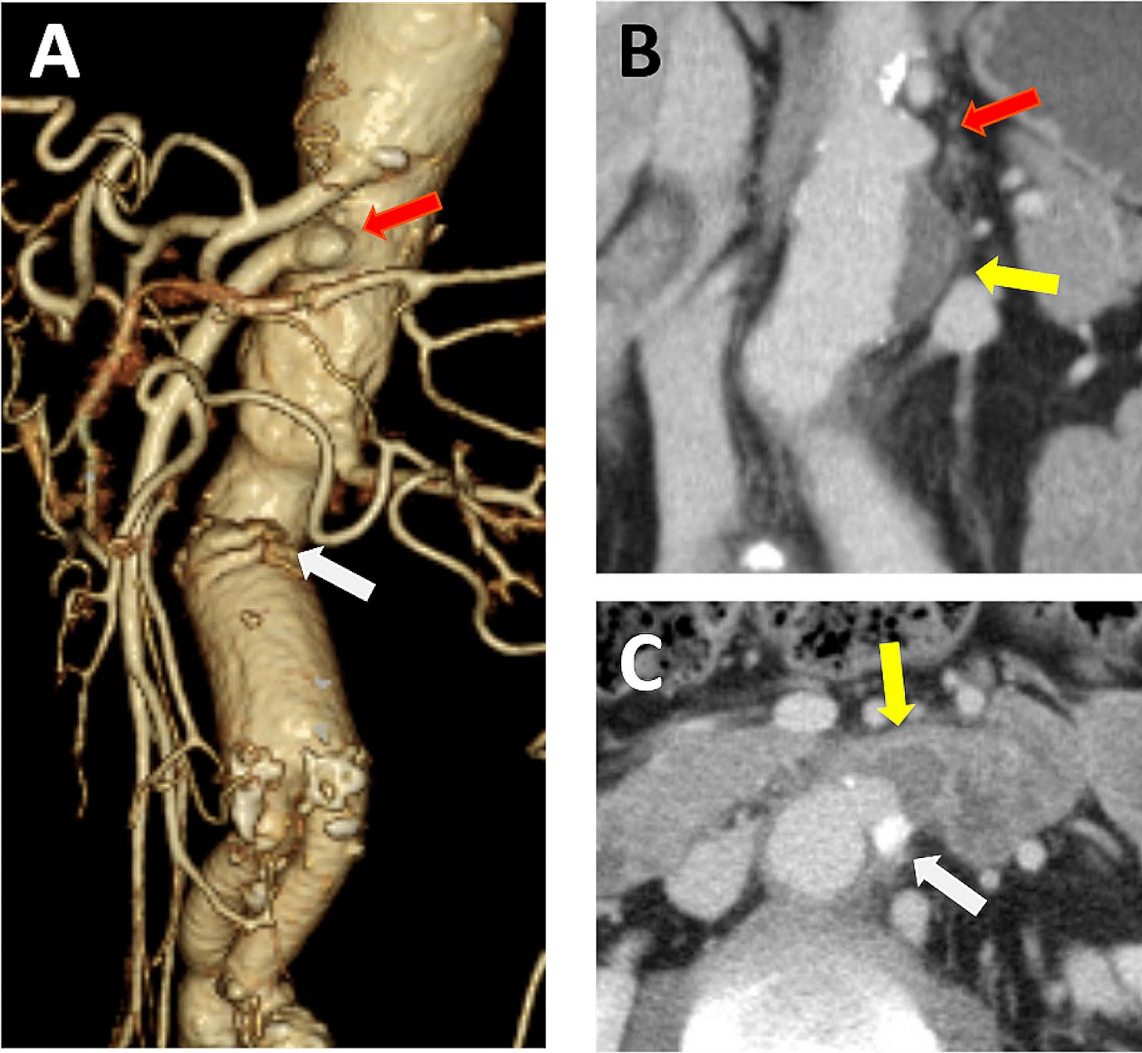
Preoperative computed tomography. A Three-dimensional view showing a pseudoaneurysm of the anastomotic site (white arrow) and a pseudoaneurysm at the superior mesenteric artery bifurcation (red arrow). B Coronal view demonstrating a pseudoaneurysm at the superior mesenteric artery bifurcation (red arrow) and atheroma on the central side of the anastomotic site (yellow arrow). C Axial view demonstrating a pseudoaneurysm of the anastomotic site (white arrow) and soft tissue that connected the horizontal part of the duodenum (yellow arrow)
